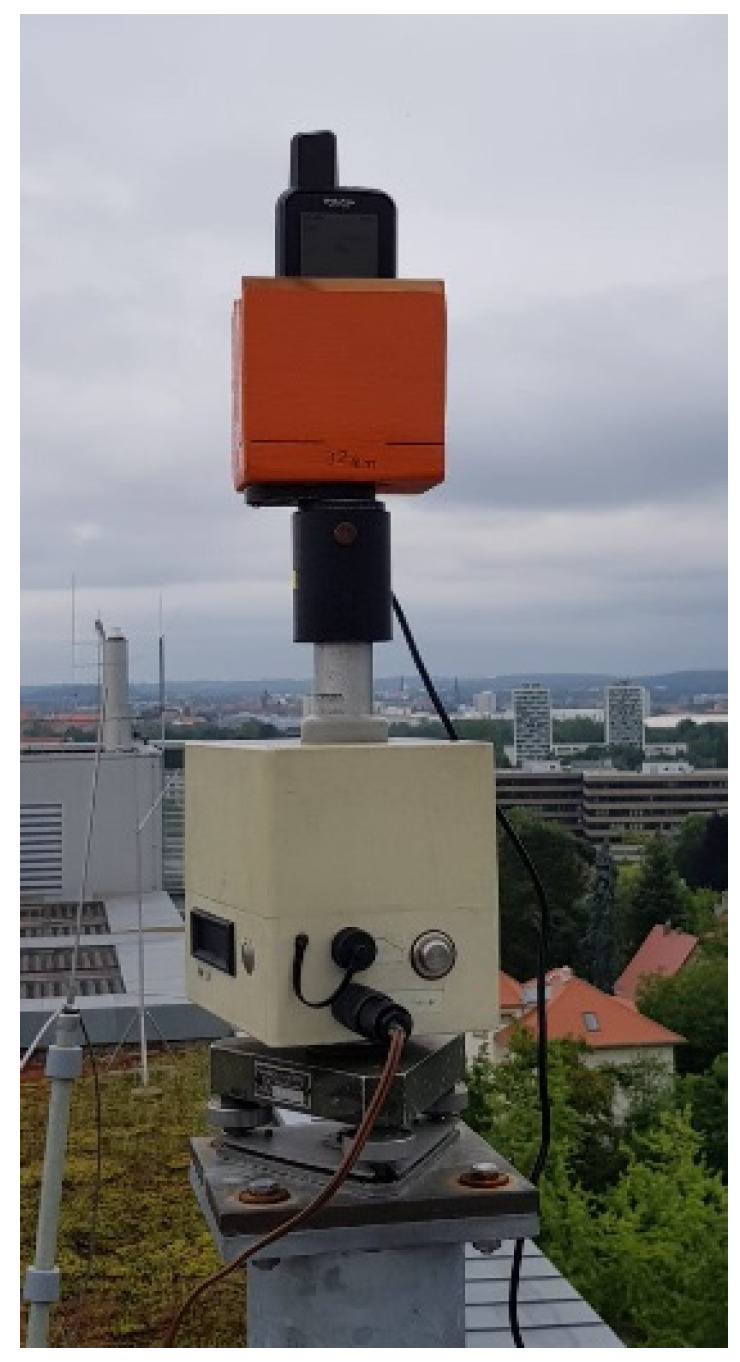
GPSMAP 66sr in its holder on top of the DRB2 rotational device, which enables observations in four azimuthal orientations per minute.
